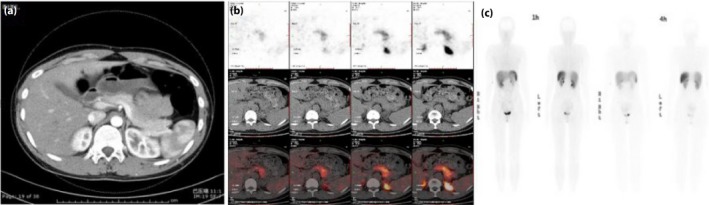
Negative results of radiological examinations. (a) Pancreatic perfusion computed tomography image of case 1. (b) 68Ga‐NOTA‐exendin‐4 positron emission tomography/computed tomography and (c) somatostatin receptor scintigraphy images with ^99m^Tc‐hydrazino nicotinamide‐octreotide of case 2.
